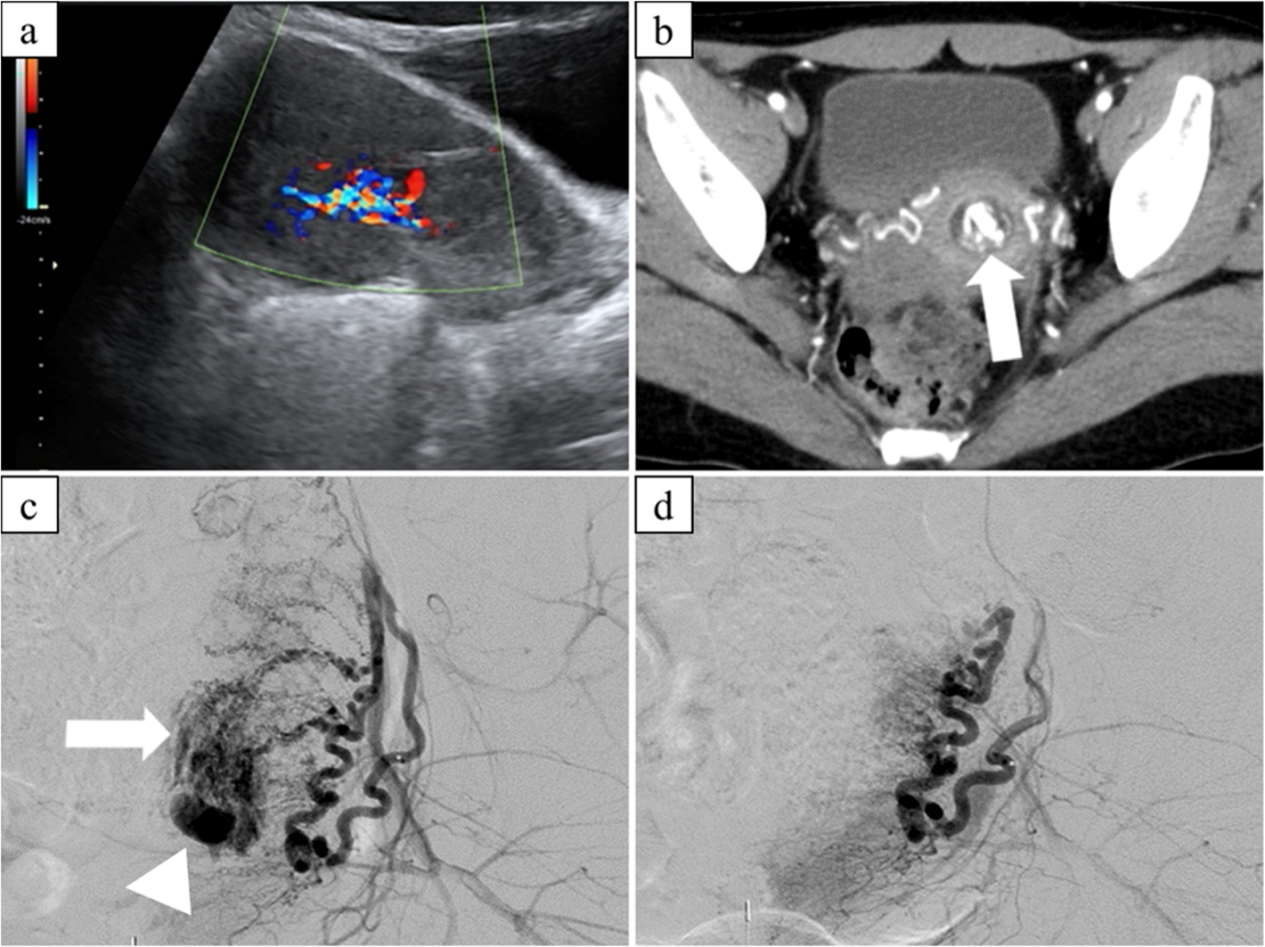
32-year-old patient with vaginal bleeding post D&C for missed abortion. a Trans-abdominal ultrasonography showing a hypervascular intrauterine mass. b Arterial-phase CE-CT showing an enhancing lesion in the uterine cavity (arrow). c Left uterine arteriogram showing a focal blush (arrow) and a pseudoaneurysm (arrowhead). d Post-embolization left uterine arteriogram confirming the disappearance of the pseudoaneurysm and focal blush
